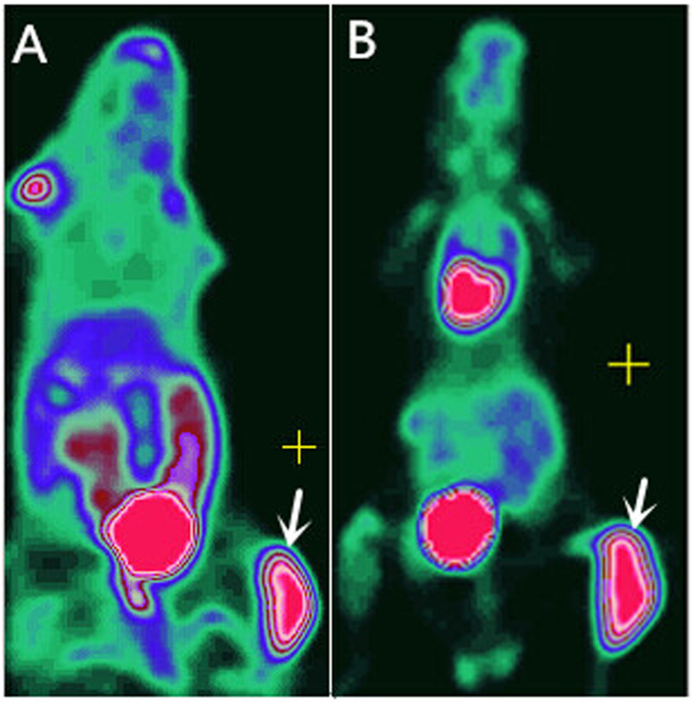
Biodistribution by whole body PET Imaging. Figure 2A, ^18^F-Alfatide PETimaging. Figure 2B, ^18^F-FDG PET imaging. Arrows pont to tumor.
